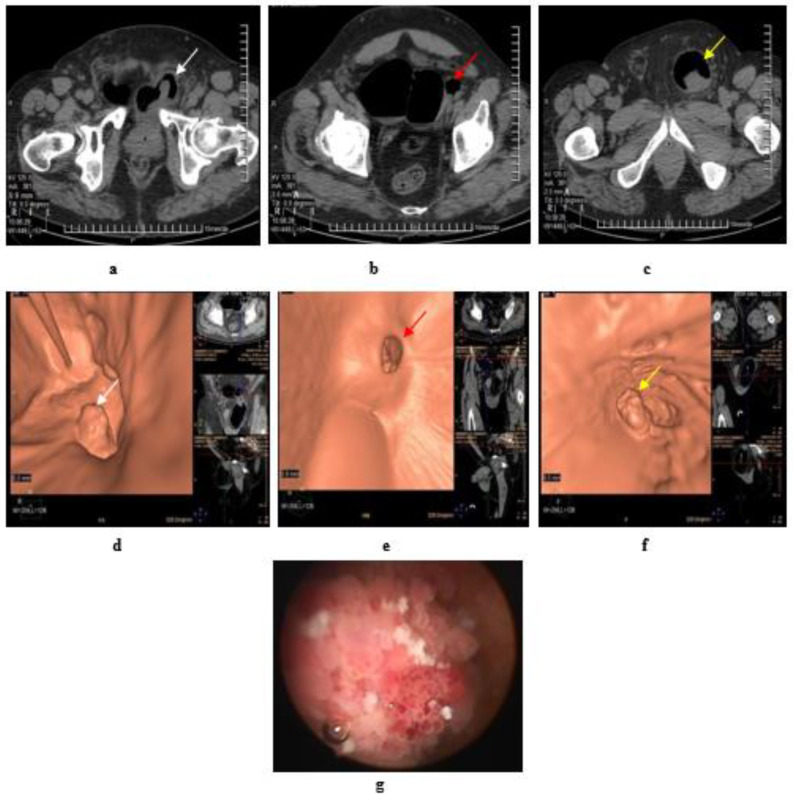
Papillary urothelial carcinoma of the bladder. (a) Axial MDCT of the pelvis after air insufflation: intravesical soft tissue mass (white arrow) at left posterolateral wall. (b) A large right bladder diverticulum with a wide neck and a small left diverticulum showed two small intradiverticular soft tissue masses (red arrow). (c) A large left inguino-scrotal hernia with a large cystocele inside and a soft tissue mass within the cystocele (yellow arrow). (d) Virtual CT cystoscopy of the intravesical mass noted at left posterolateral bladder wall, that was referred to at (a) (white arrow). (e) Virtual CT cystoscopy of two small soft tissue masses inside a small left side diverticulum, that was referred to at (b) (red arrow) (f) Virtual CT cystoscopy of a large soft tissue mass at the cystocele arising from its posterior wall, that was referred to at (c) (yellow arrow). (g) Conventional cystoscopy confirms the presence of intravesical masses.
